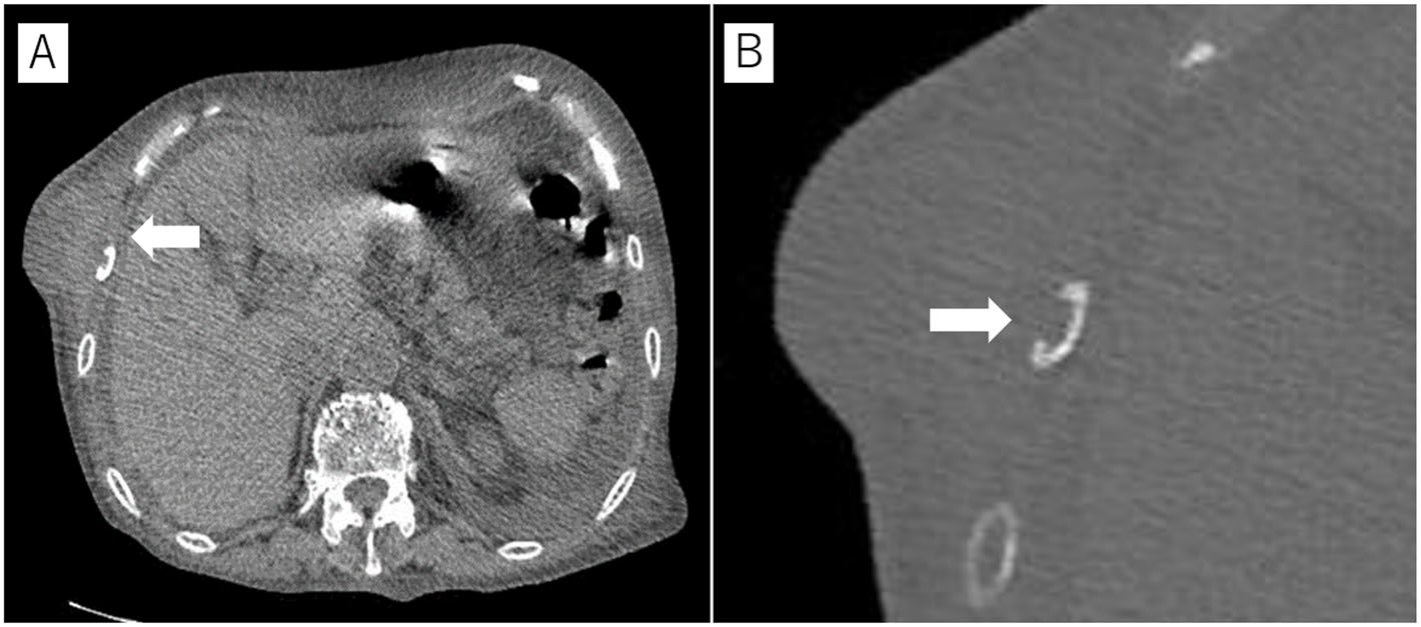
Computed tomography scan. a A mass in the chest wall, approximately 65 × 30 mm. b Right 8th rib with osteolytic change
